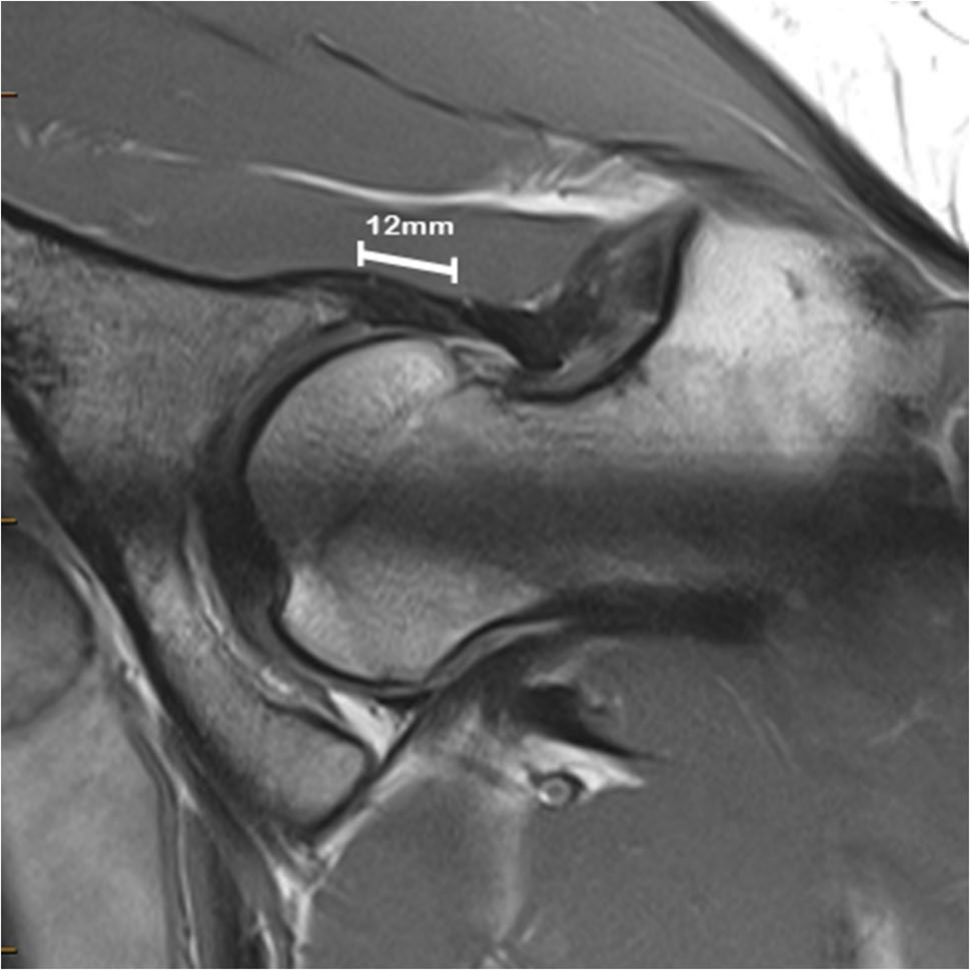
Radial PD MR image in a 21-year-old female with clinical microinstability, demonstrating elongated labrum superior labrum
